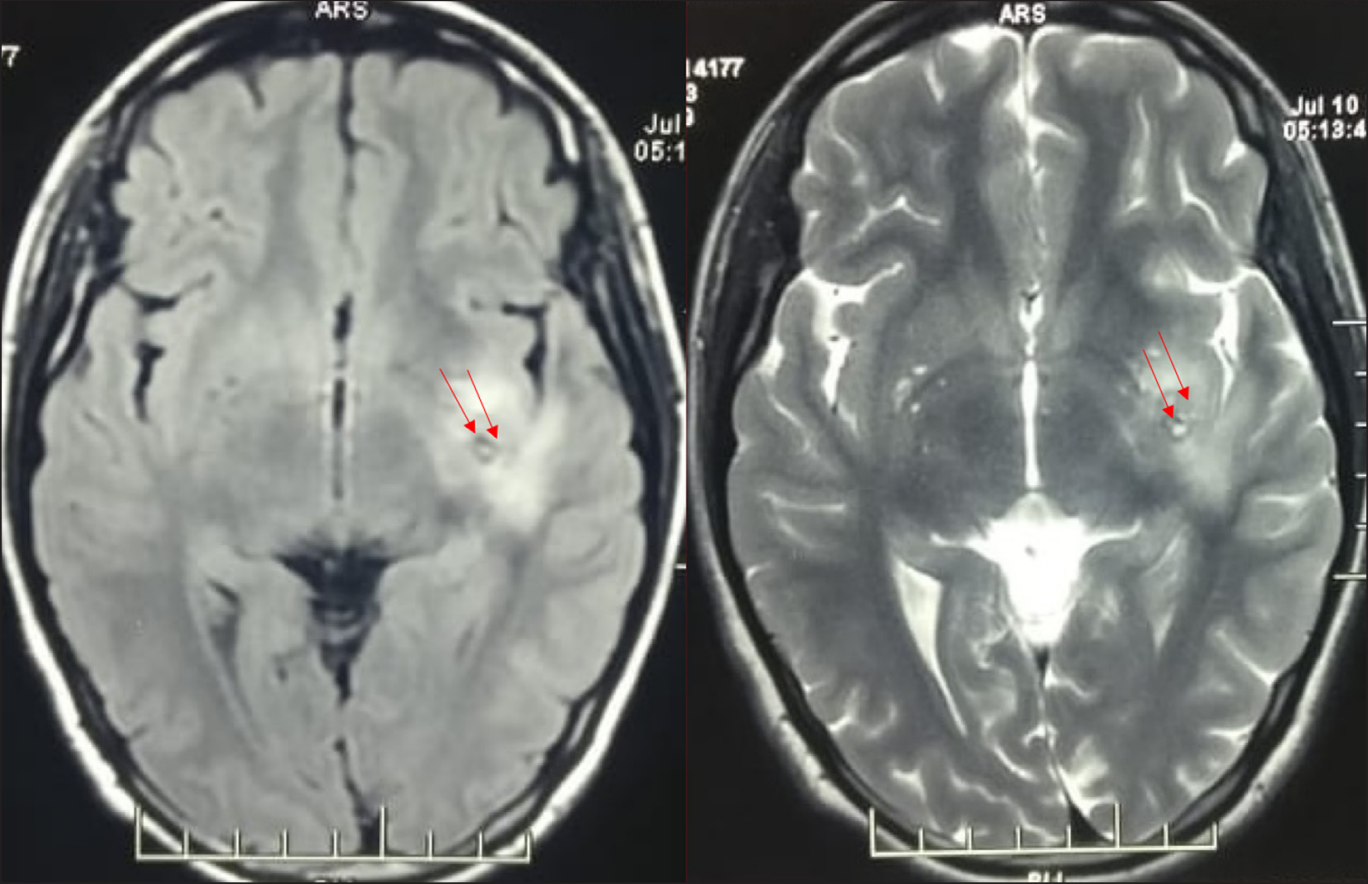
MRI of the brain reveals a cystic lesion with an eccentric scolex and surrounding edema located near left thalamic region. Clinically, the patient presented with right-sided hemichorea (Video).
